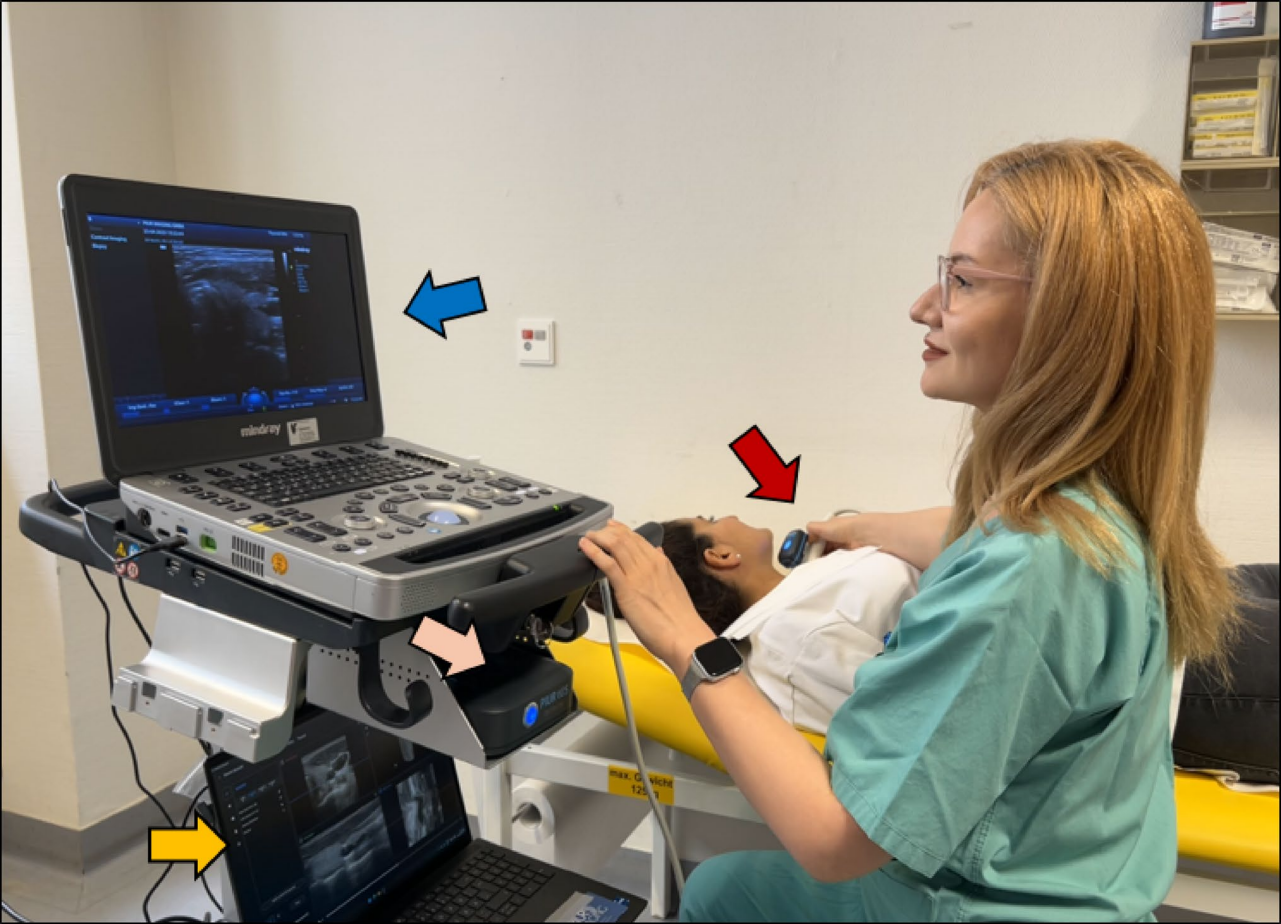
Use of AI-software during a preoperative thyroid and neck ultrasound
